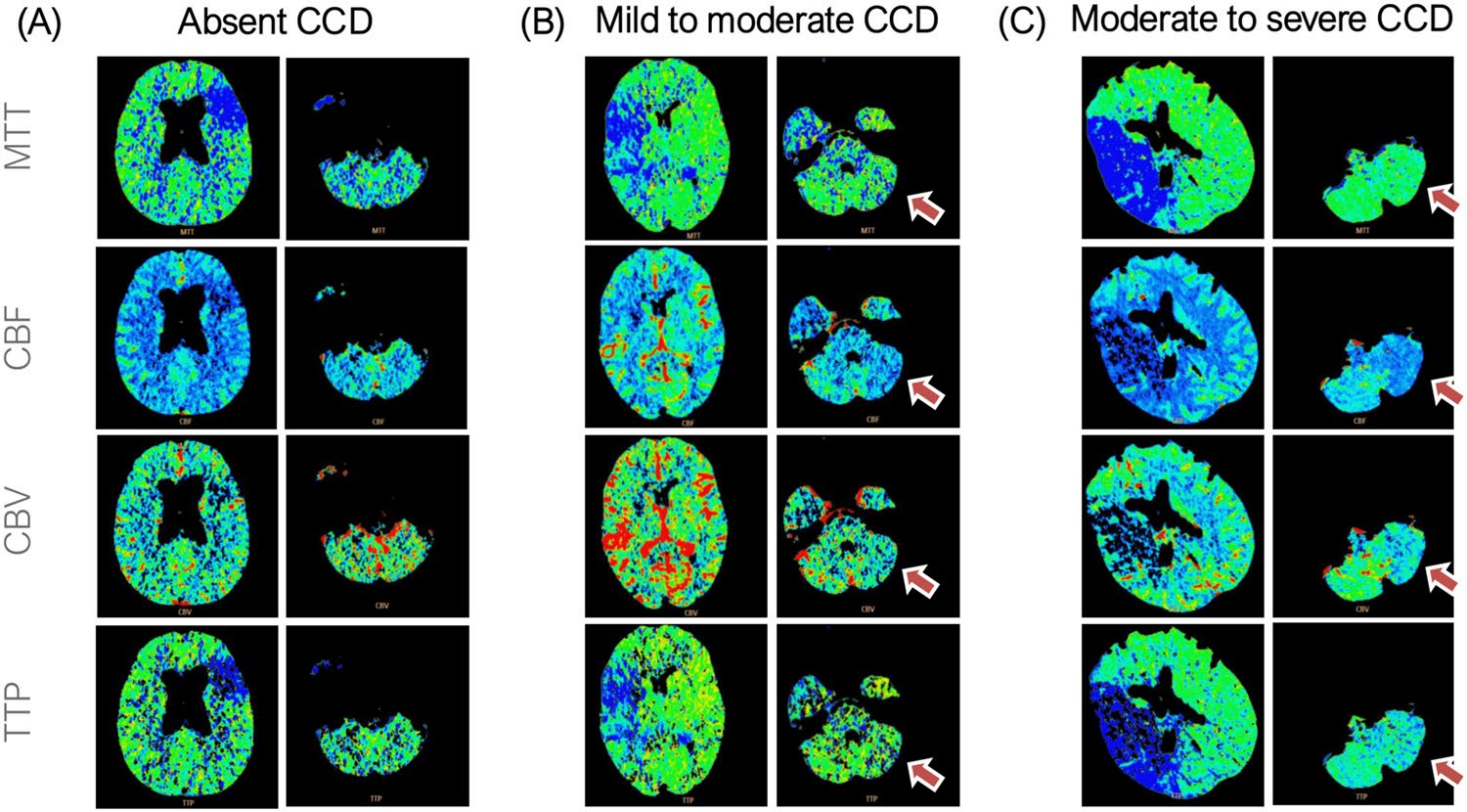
Representative cases of absence of CCD, mild to moderate and moderate to severe CCD. A A 77 year-old man presented to the emergency department two hours after symptom onset with right-hand weakness and motor aphasia (baseline NIHSS: 3). Imaging revealed an occlusion of the left M2 segment of MCA, and CT perfusion demonstrated a hypoperfused volume (MTT) of 33 mL without evidence of CCD. The patient received intravenous thrombolysis and was discharged with a NIHSS score of 0. At the 3 month follow-up, the mRS was 0, indicating complete recovery without residual deficits. B A 44-year-old woman presented to the emergency department one hour after the onset of left-sided sensorimotor symptoms (baseline NIHSS: 16). Imaging revealed an occlusion of the right M1 segment of MCA, and CT perfusion demonstrated a hypoperfused volume (MTT) of 93 mL with evidence of mild-to-moderate CCD. The patient underwent intravenous thrombolysis followed by mechanical thrombectomy, achieving complete recanalisation (mTICI 3). She was discharged with a final NIHSS score of 0, and at the 3 month follow-up, the mRS was 0, indicating full recovery without residual deficits. C A 74 year-old woman presented to the emergency department two and a half hours after the onset of left hemiplegia and dysarthria (baseline NIHSS: 22). Neuroimaging revealed occlusion of the right internal carotid artery (ICA) extending to the M1 segment of the middle cerebral artery (MCA). CT perfusion demonstrated a hypoperfused volume (MTT) of 190 mL with evidence of moderate-to-severe CCD. The patient underwent intravenous thrombolysis followed by mechanical thrombectomy, achieving partial reperfusion (mTICI 2a). She was subsequently transferred to a rehabilitation facility with an NIHSS score of 15. At the 3 month follow-up, the mRS was 5, with persistent motor deficits
